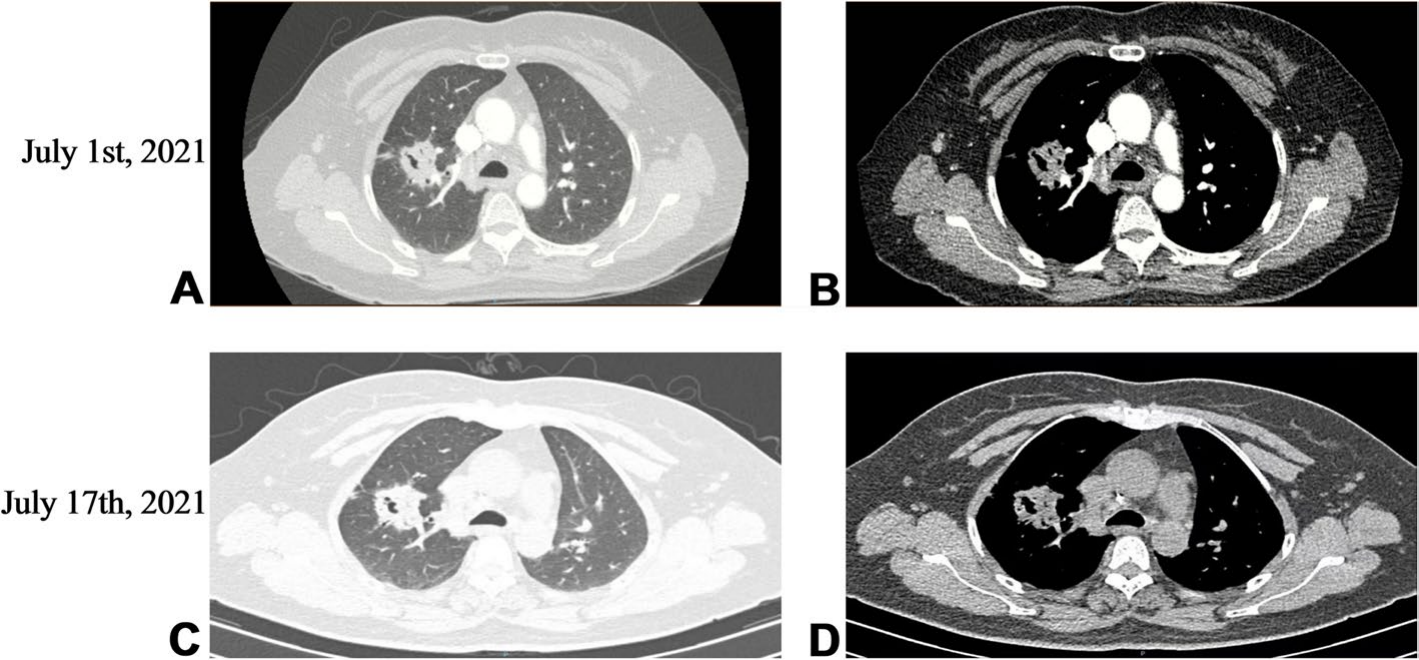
Chest CT at different time points. July 1st, 2021 Chest enhanced CT showed irregular soft-tissue density mass in the upper lobe of the right lung, and the bronchial branch of the upper lobe of the right lung was invaded and narrowed, which suggested a high possibility of lung cancer. There were also several small punctate calcification foci in the left lung (A). Slight calcification in mediastinum and left hilar lymph nodes (B). July 17th, 2021 After antifungal therapy, CT showed irregular soft tissue density shadow in the upper lobe of the right lung with cavity formation, which was considered to be lung cancer, with little change from the CT result before treatment (C, D)
